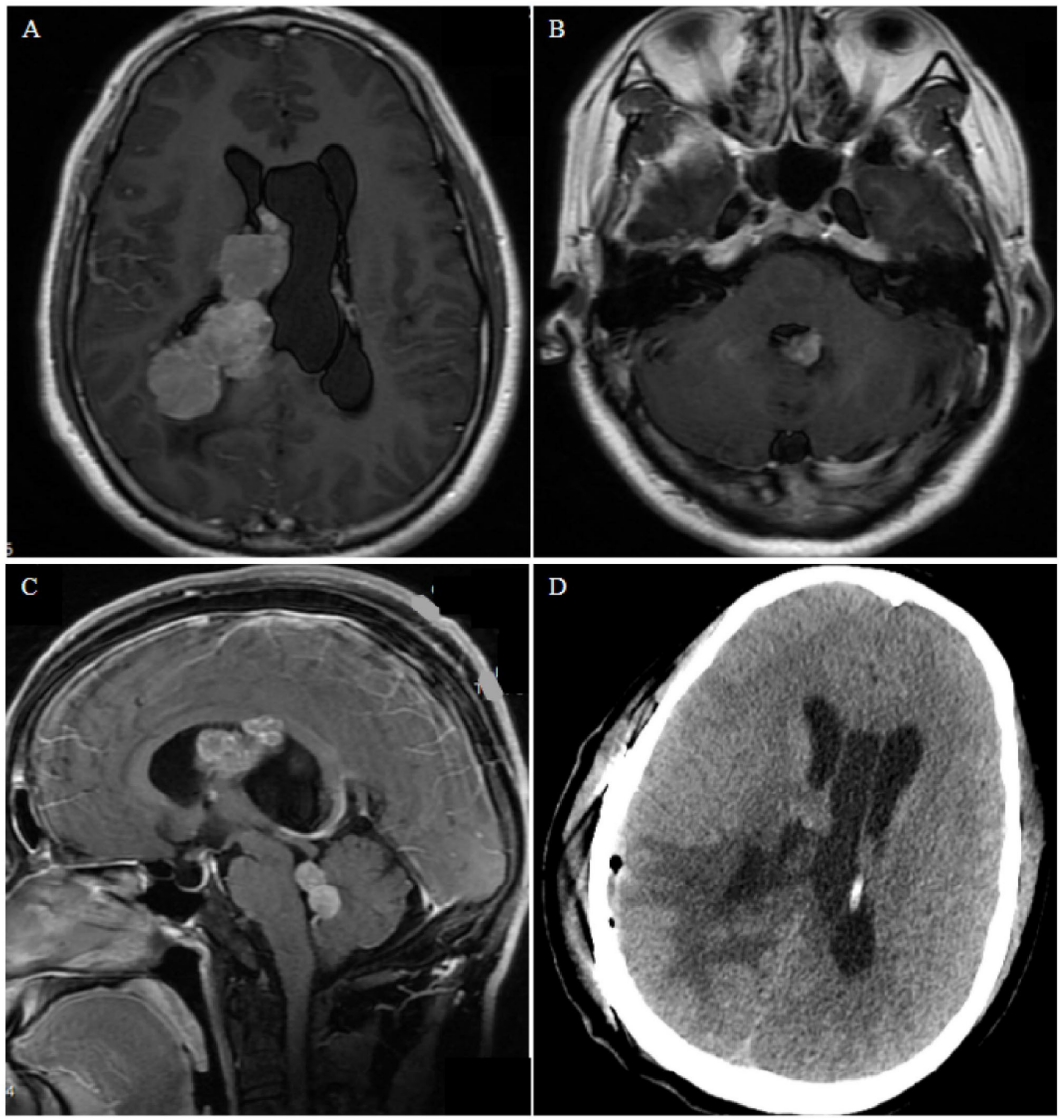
Case 2. (A,B) Contrast-enhanced T1-weighted axial images show nodular bead-like lesions in the right and fourth ventricles. (C) Sagittal magnetic resonance images showing multiple lesions occupying the midbrain aqueduct and fourth ventricle. (D) Postoperative computed tomography shows edema of brain tissue and no bleeding in the operative cavity.
